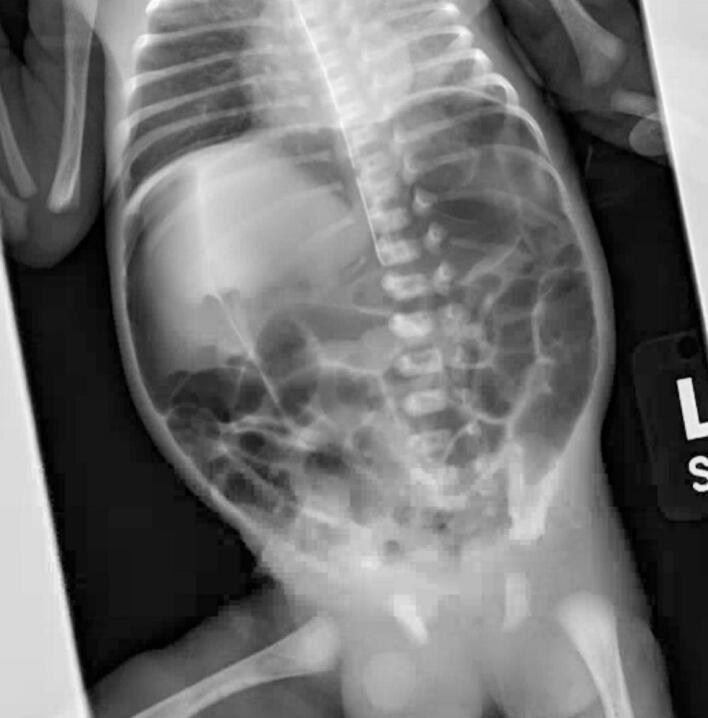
Abdominal radiograph demonstrating significant pneumoperitoneum.
